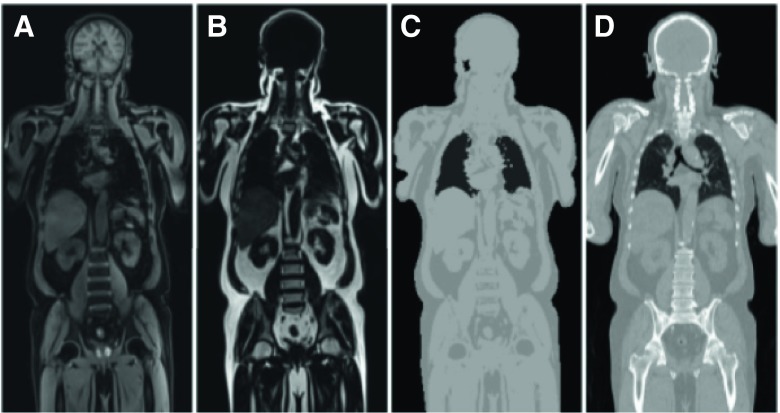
Dixon MRI-based attenuation correction used for PET-MRI. Shown are the Dixon water (A) and fat (B) images. C. MRI-based attenuation map was generated by combining water and fat images. D. CT of same patient. Adapted from Yoo et al. [84] (open access)
